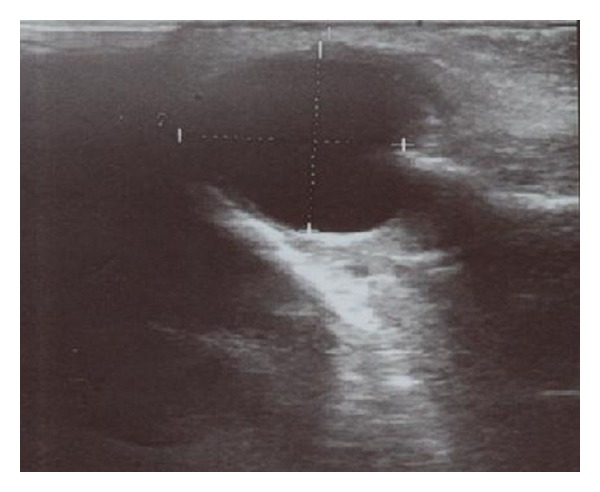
Ultrasonography image showed 1.5 cm diameter soft tissue mass in nasolabial fold.
